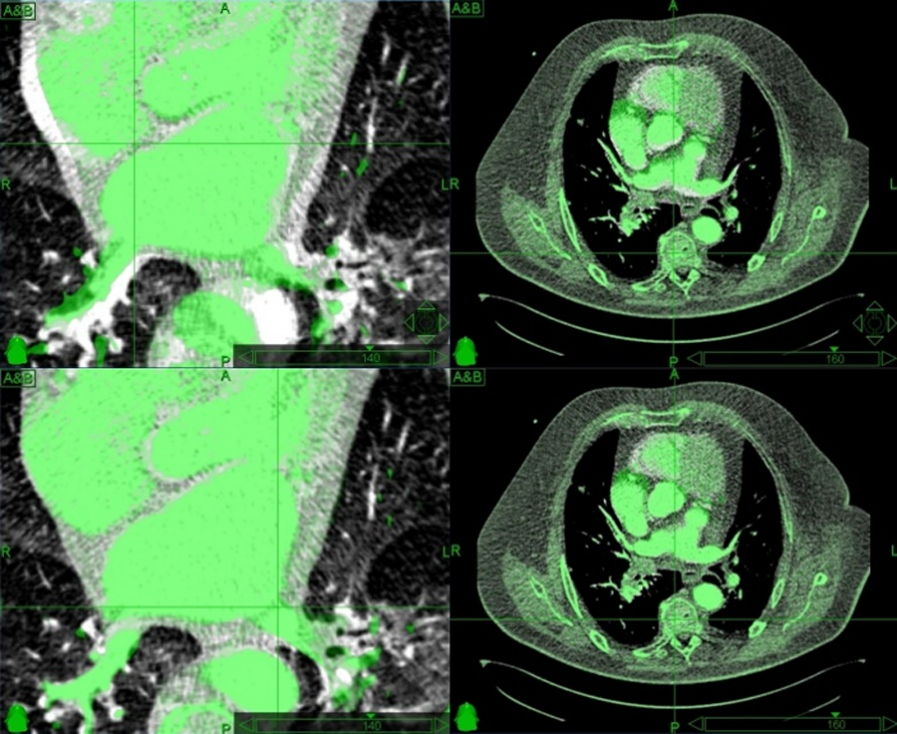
Alignment and deformation modeling of an example patient CT Differential motion over the respiratory cycle can be seen in the lower left and right pulmonary vein after alignment to the right atrial septum (top left). Maximum cardiac motion was approx. 1 cm in the right atrial septum (top right). Deformation modeling of respiratory (bottom left) and cardiac (bottom right) cycle was sufficient.
